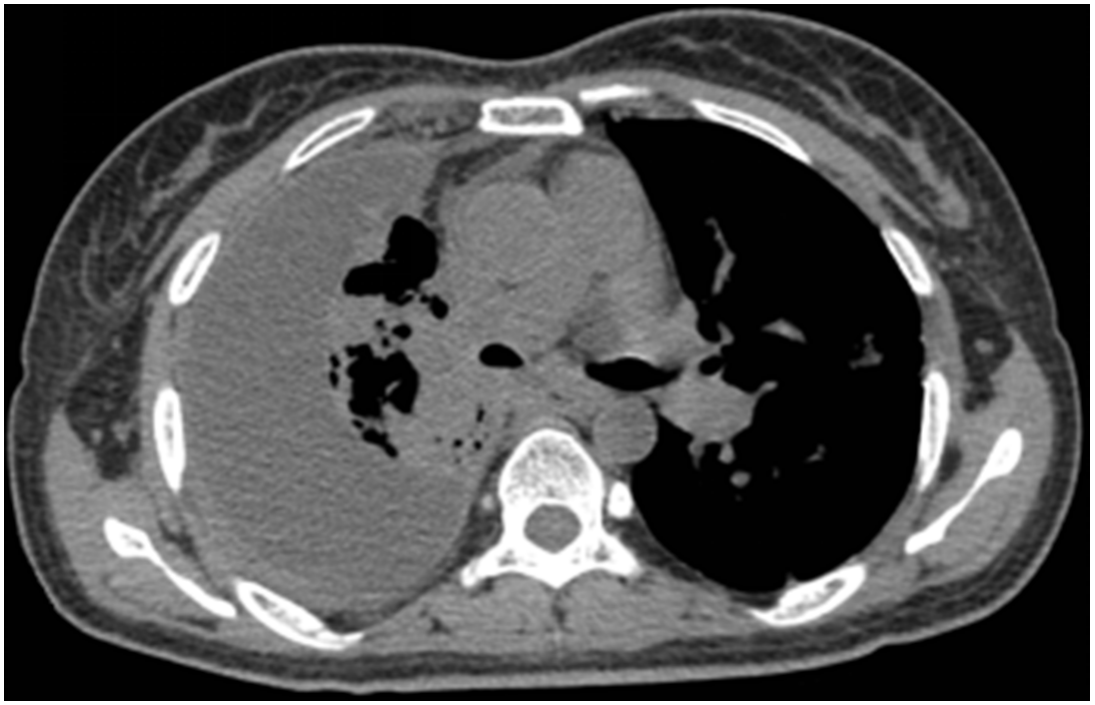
CT scan showed decreased pleural effusion with lung recruitment
